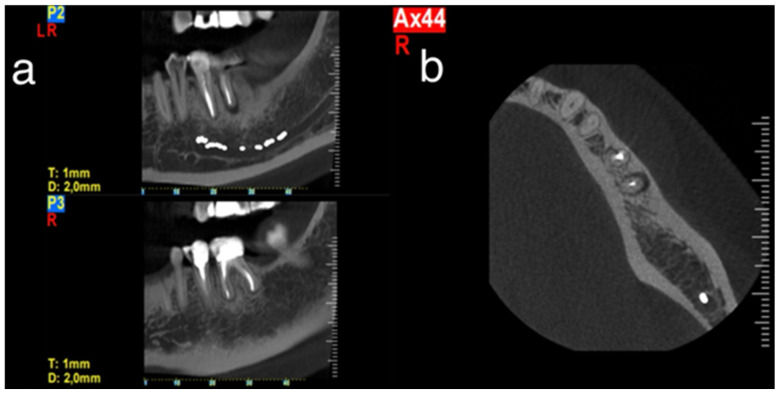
CBCT images of tooth #36 confirming localized pathology of the mesial root. (a) Coronal view, radiolucency around the mesial root of tooth #36. (b) Axial view, a very localized, angular, fissure-like radiolucency on the buccal surface of the mesial root of tooth #36.
